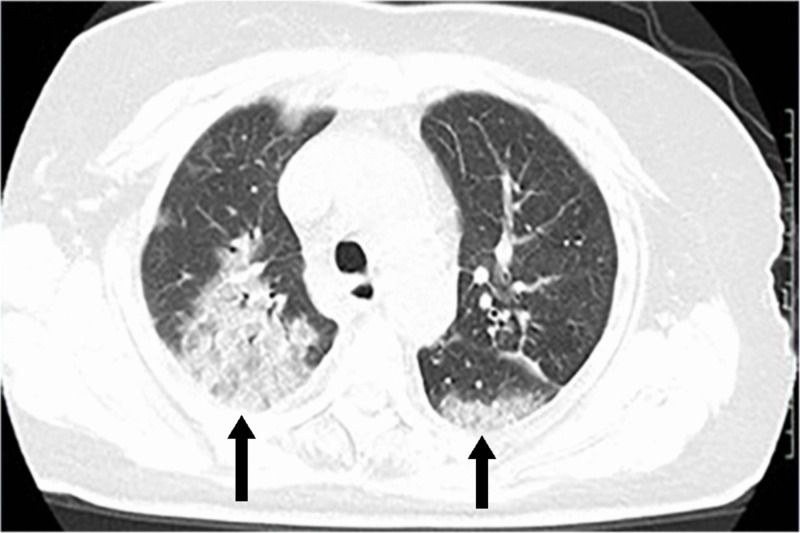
CT of the chest in a COVID-19 patient Axial CT of the chest showing GGO and bilateral posterior opacities with a paving pattern. Photo courtesy of Salehi et al [35]. CT, computed tomography; COVID-19, coronavirus disease of 2019; GGO, ground-glass opacities
